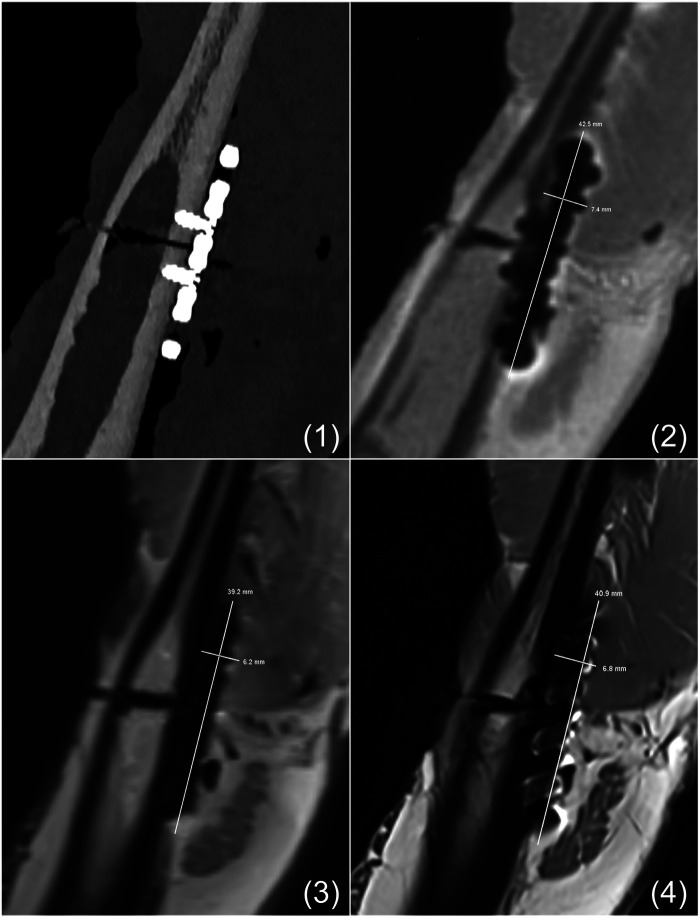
Quantification of artifact extent. Sample images for artifact quantification, from left to right and from top to bottom: (1) Gold standard photon-counting CT, (2) UTE, (3) SEMAC, and (4) WARP. In an exploratory manner, the length and width of the metallic artifacts caused by plate osteosynthesis were measured and subsequently artifact area was calculated by assuming rectangular shape. CT, computed tomography; MRI, magnetic resonance imaging; UTE, ultrashort echo time; SEMAC, slice encoding for metal artifact correction; WARP, vendor-specific artifact reduction techniques (Siemens Healthineers, Forchheim, Germany).
